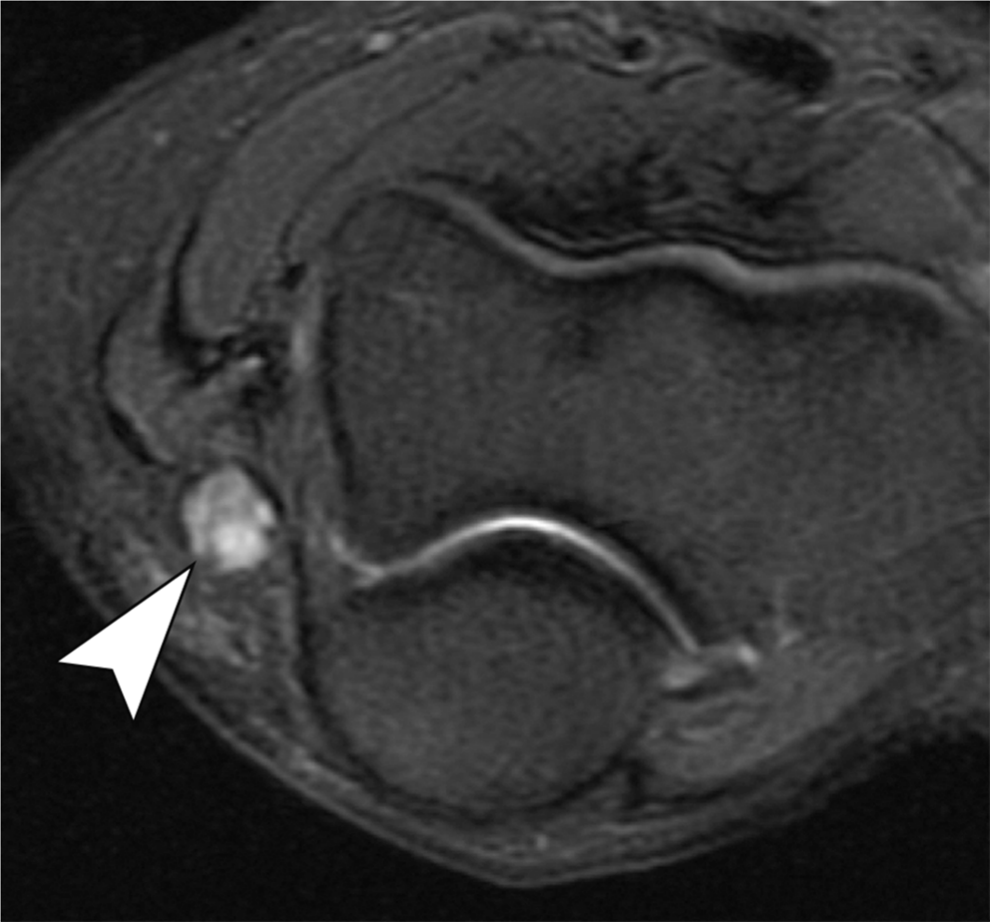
Ulnar neuropathy. Axial T2-weighted image with fat saturation. Thickening and increased signal of the ulnar nerve with loss of the regular fascicular pattern (arrowhead)
